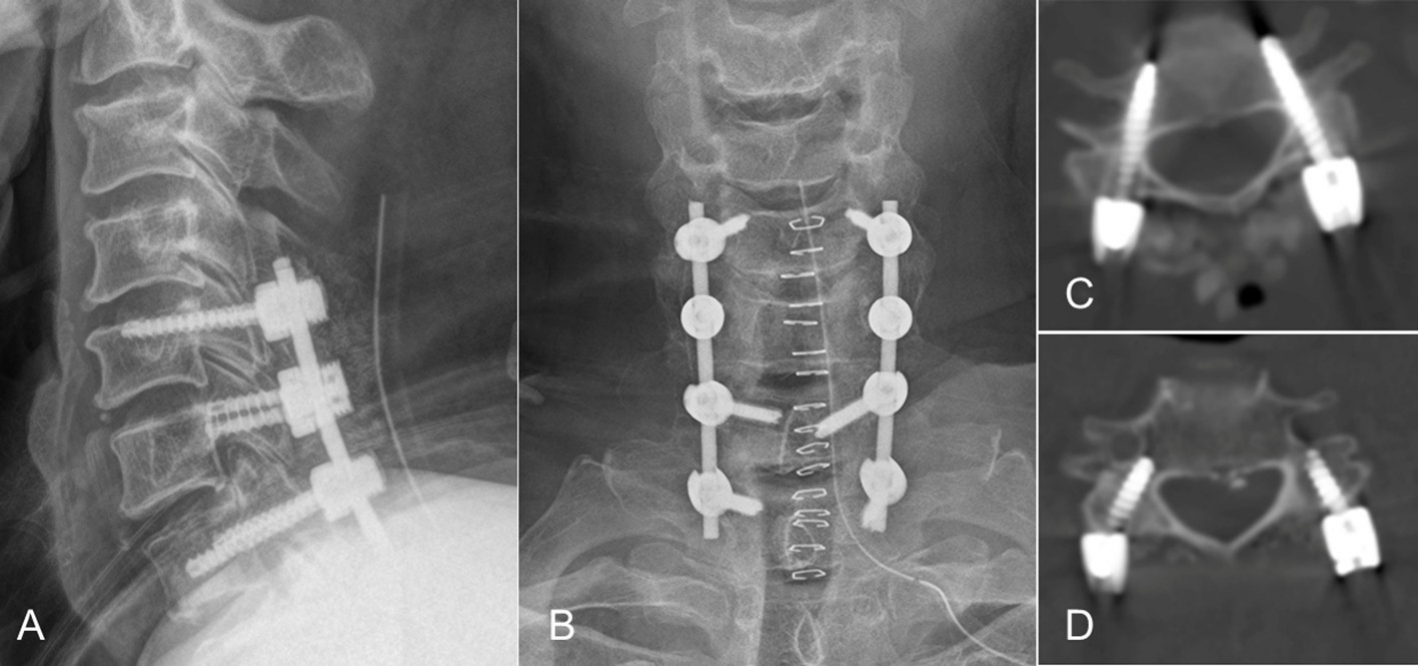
(A) (B) Postoperative radiography showing alternative usage of CPA and PVFS. CPS was employed at C5/7 and PVFS at C6. The depth of PVFS could be observed on a lateral image. The end point was just past the posterior wall of the body at C6. A-P image shows the adaptability of PVFS: no need of additional connecting rods. (C) Axial computer tomography image of C6. (D) PVFS at C6 was. The ideal location of PVFS was on the right. On the left side, PVFS was laterally located and touched the vertebral foramen but remained safe if the screw stopped just anterior of the posterior wall of the vertebral body.
